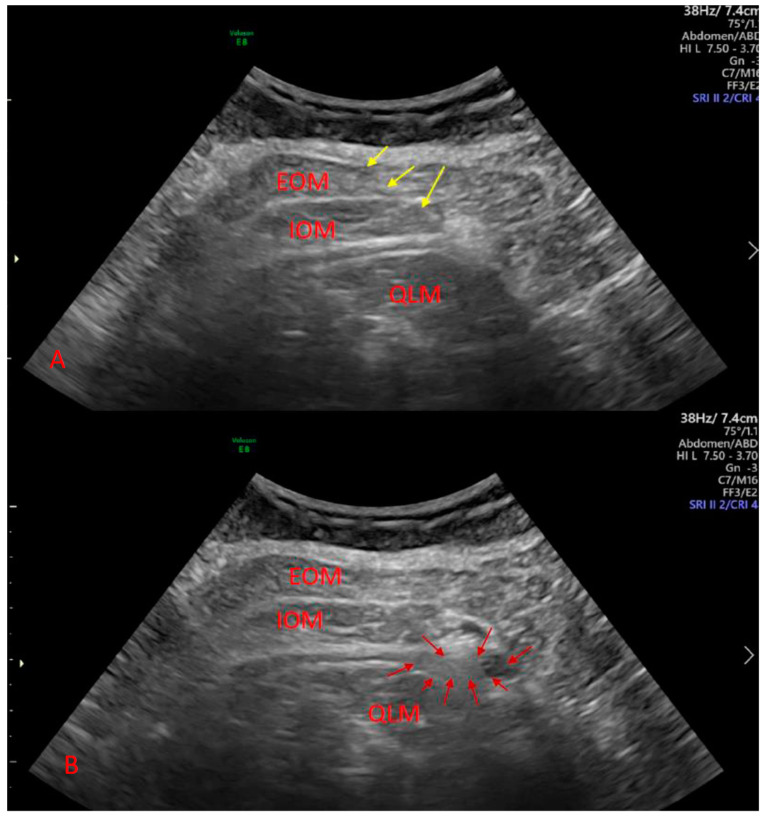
Ultrasound-guided quadratus lumborum block.
